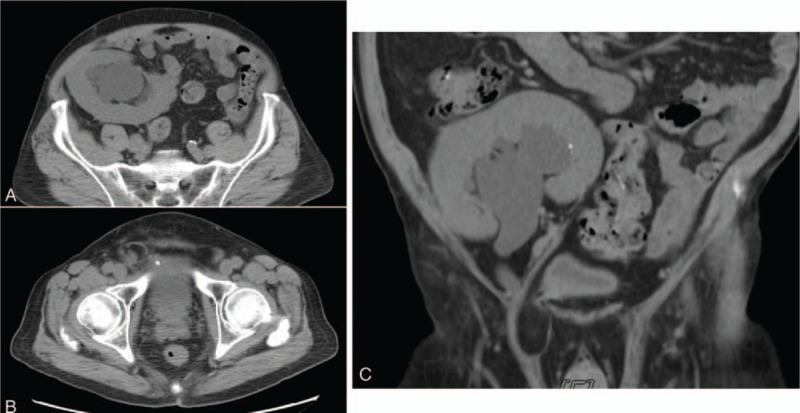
CT scan. (A) The renal hilum of the transplanted kidney is seen facing upwards. (B) The ureter extends superficially downwards towards/into the bladder; however, is compressed by the hernial sac and is therefore entrapped in the inguinal canal. (C) Ureter is compressed by the inguinal hernia.
